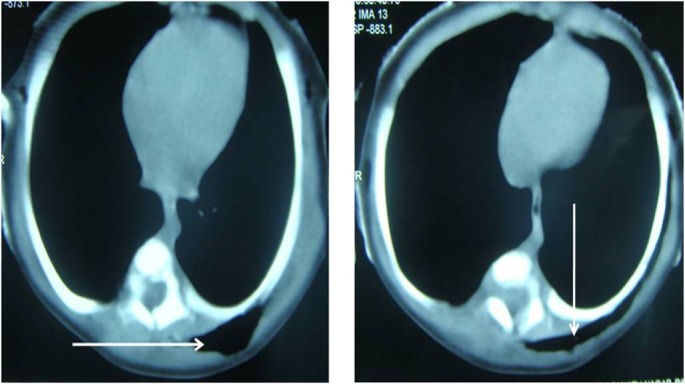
Figure 2: CT scan of the chest showing subcutaneous emphysema (arrows) in the left lower posterior thoracic wall.
